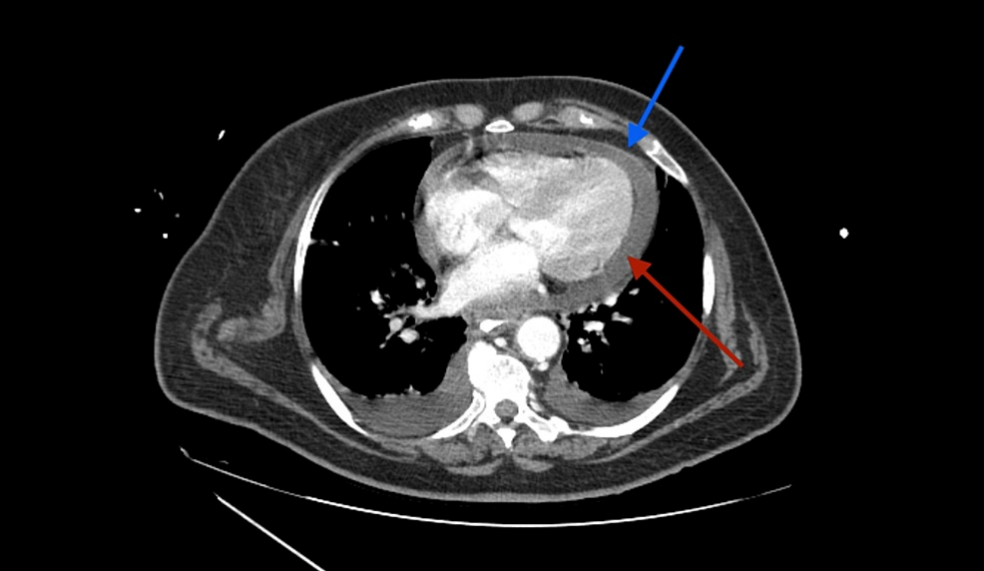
Imagiological signs of acute pericarditis Contrast-enhanced thoracoabdominal CT highlighting a small- to moderate-volume pericardial effusion (red arrow) associated with enhanced pericardial thickening after contrast in favor of pericarditis (blue arrow) CT: computed tomography
